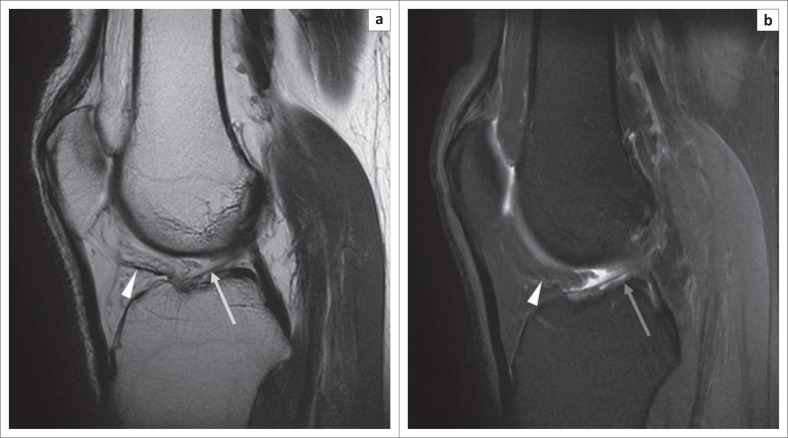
Magnetic resonance imaging of a normal infrapatellar plica: sagittal T2-weighted image (T2WI) (a) and sagittal proton density fat-saturated (PDFS) image (b) revealing the normal infrapatellar plica as a thin low-signal intensity structure anterior to anterior cruciate ligament in the inter-condylar notch (arrow) and in Hoffa’s fat pad (arrow head).
